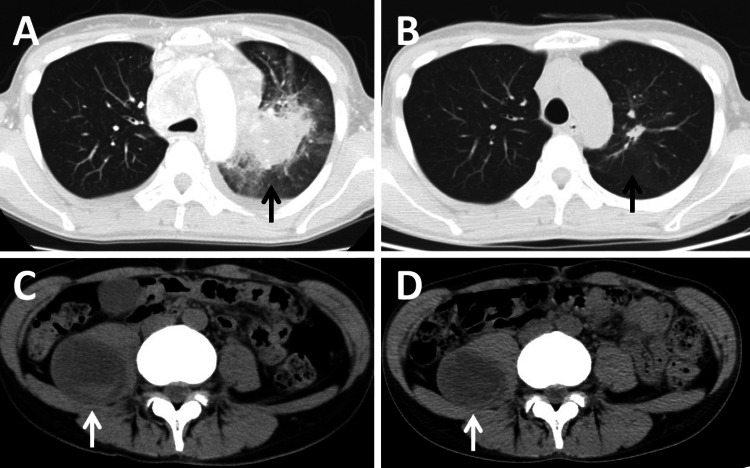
CT scans before and after alectinib treatment A and B, and C and D are CT images of the chest, and the abdomen, respectively. A and C represent CT images prior to the start of alectinib treatment, while B and D are images taken 44 days after treatment initiation. Arrows highlight tumor locations.
